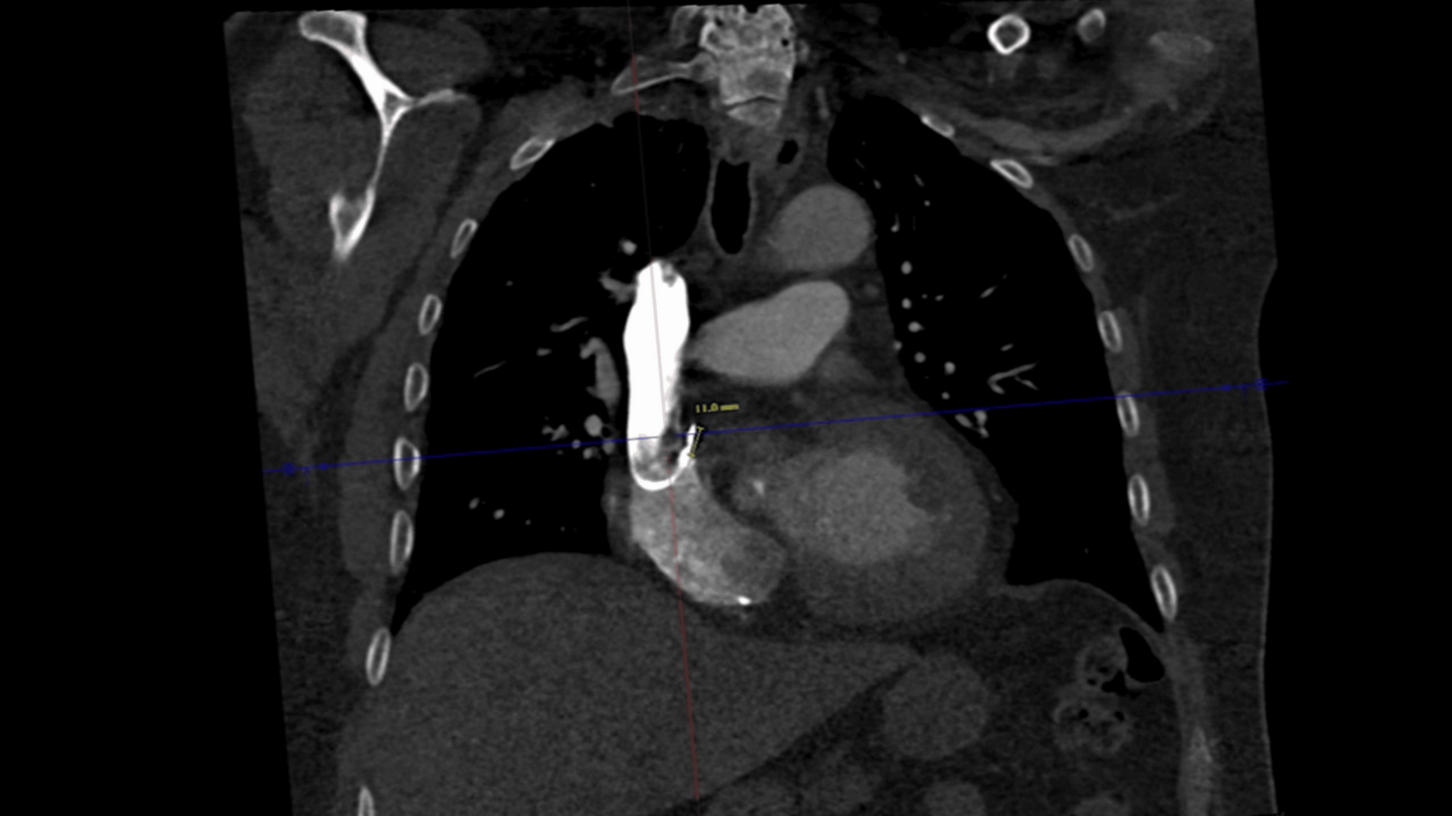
CT imaging of the chest revealed migration of the right atrial pacemaker lead. CT imaging of the chest revealed migration of the right atrial pacemaker lead into the medial portion of the proximal right atrium, positioned posterior to the aorta at the right atrial-superior vena cava junction.
